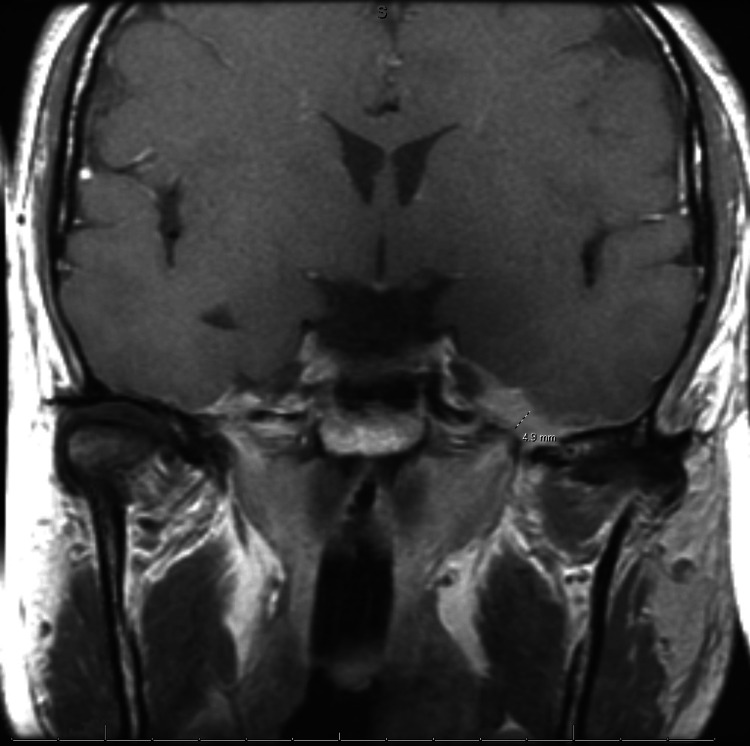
Coronal T1-weighted MRI scan showing 4.9 mm smooth dural thickening and enhancement (dotted label) consistent with IHP, as opposed to GPA which typically displays local bone destruction at the base of the skull and vascular inflammation. MRI: Magnetic resonance imaging; IHP: Idiopathic hypertrophic pachymeningitis; GPA: Granulomatosis with polyangiitis
